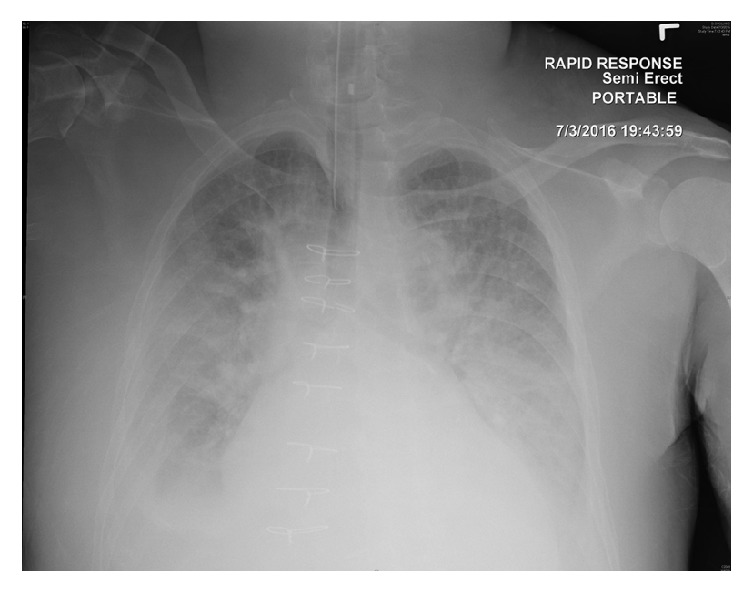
Portable chest radiograph on day 2 following intubation for respiratory distress showing worsening bilateral opacities and hilar congestion.
